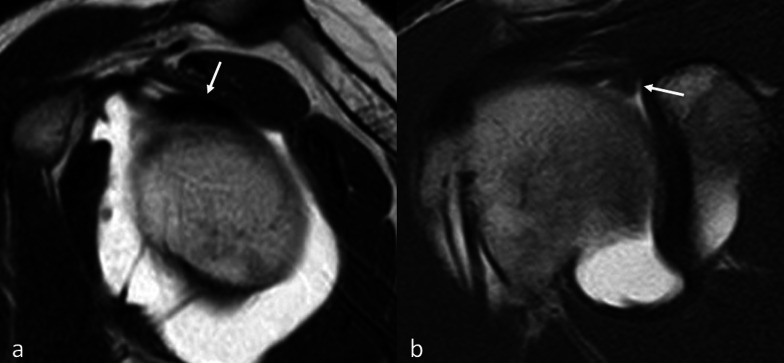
a MR arthrography, sagittal SE T1-weighted. In ADH-PM anatomical variant, long head biceps tendon is partially medially to the inferior surface of the supraspinatus (arrow). b Same patients as in a. MR arthrography, coronal SE T1-weighted. ADH-PM anatomical variant is associated with SLAP lesion type II (arrow)
